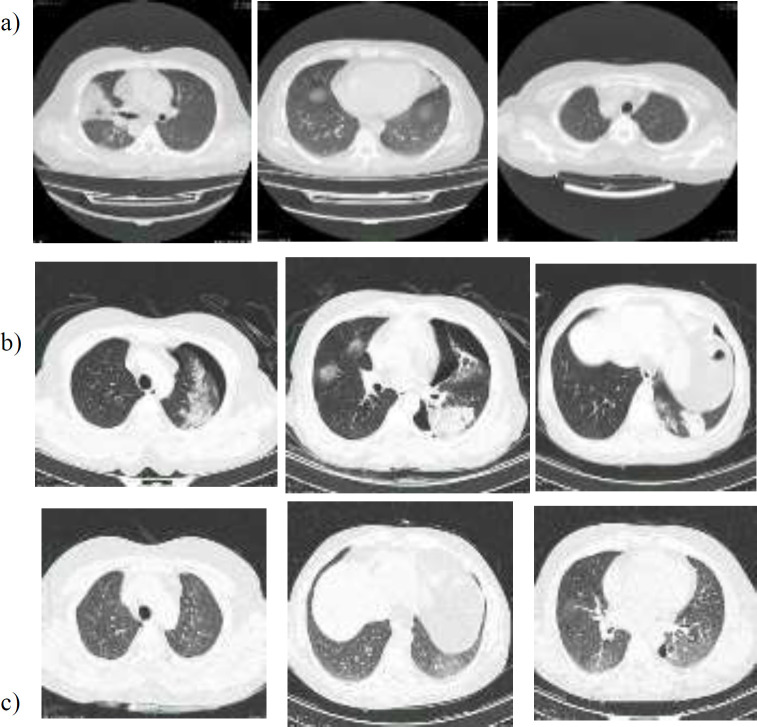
CT scan of a man with spontaneous pneumothorax due to the COVID-19
